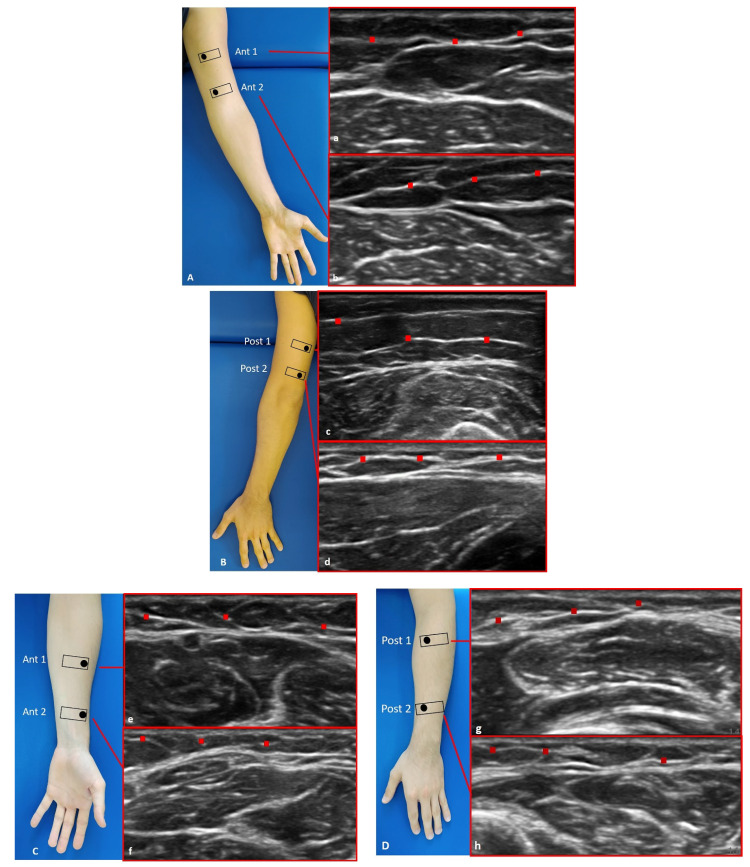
Ultrasound (US) images of the superficial fascia of: the anterior region of the arm (A) and of the forearm (C); the posterior region of the arm (B) and of the forearm (D). Anterior regions (A,C) at levels Ant 1 (a,e) and Ant 2 (b,f). Posterior regions (B,D) at levels Post 1 (c,g) and Post 2 (d,h). Probe: black rectangle; Red dashes: superficial fascia.
